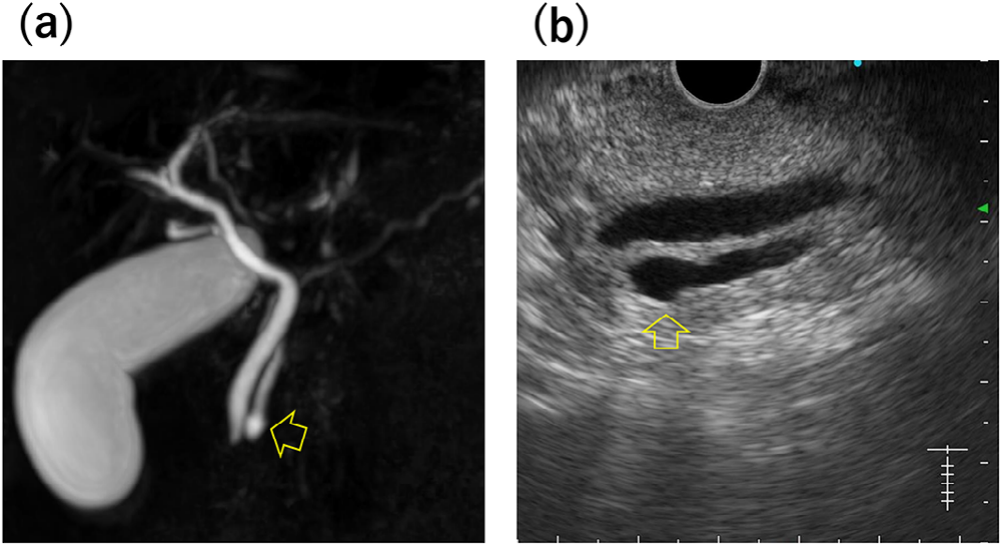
(a) Magnetic resonance cholangiopancreatography (MRCP) image reveals cystic dilatation of the main pancreatic duct above the papilla. (b) Endoscopic ultrasound (EUS) image shows focal cystic dilation of the pancreatic duct with a diameter of 5.7 mm at the pancreatic head. Arrows indicate the focal cystic dilatation area.
